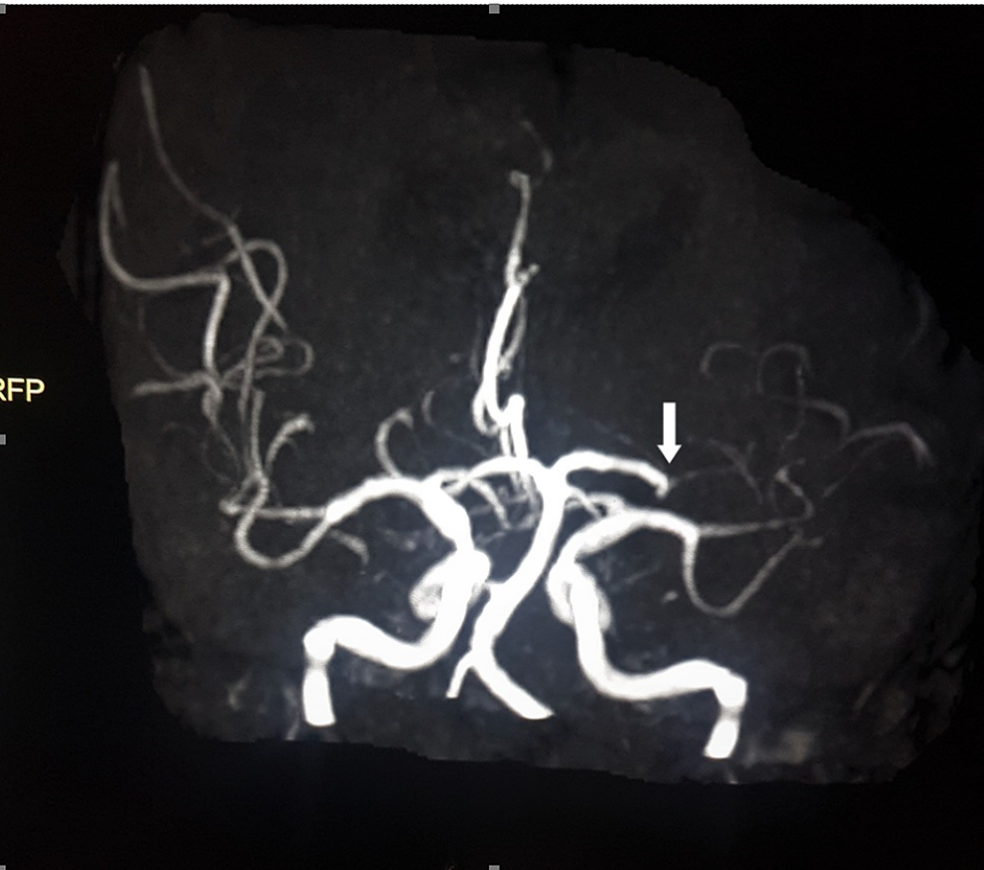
MRI angiogram of the circle of Willis showed occlusion of the left middle cerebral artery (white arrow)
